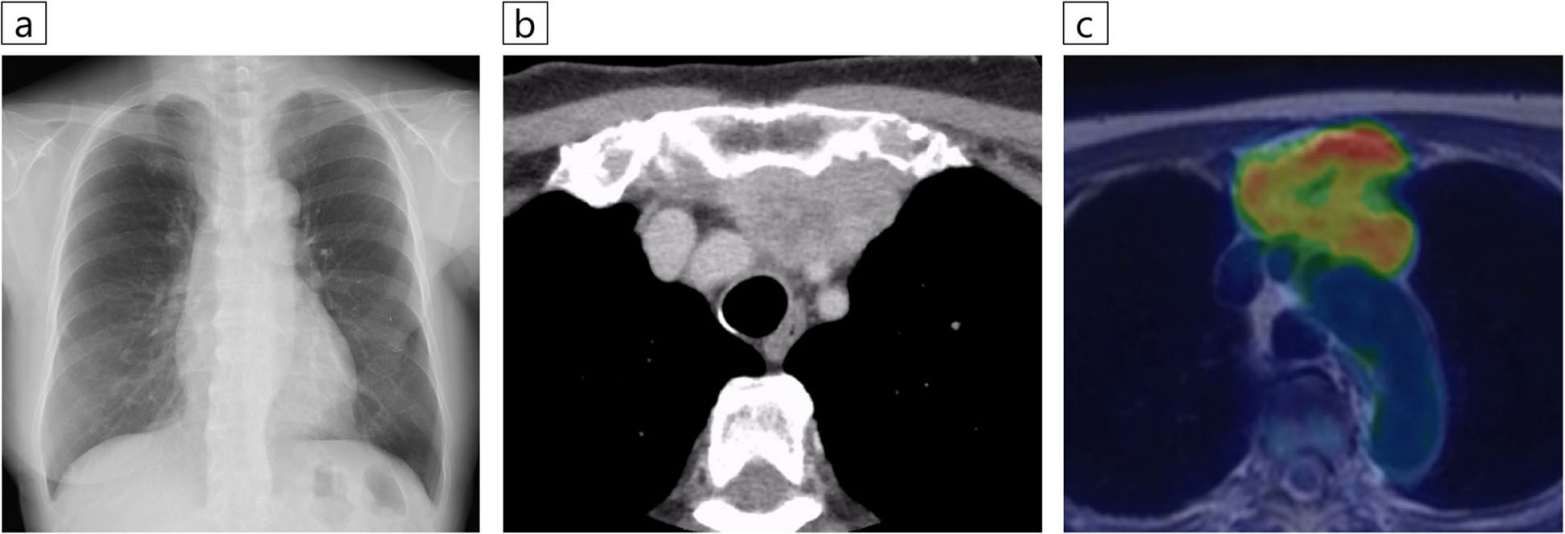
Radiographic imaging findings. a Chest X-ray showed an abnormal opacity in the mediastinum at the patient’s first visit to our hospital. b Chest contrast-enhanced computed tomography showed a mass with a maximum diameter of 5.2 cm in the anterior mediastinum. The lesion was extensively in contact with the sternum on the ventral side and partly in contact with the trachea on the dorsal side, between the brachiocephalic and the left common carotid arteries. c ^18^F-fluorodeoxyglucose-positron emission tomography (FDG-PET) showed high FDG uptake (maximum standardized uptake value was 6.71) in the anterior mediastinal mass and sternal infiltrate
